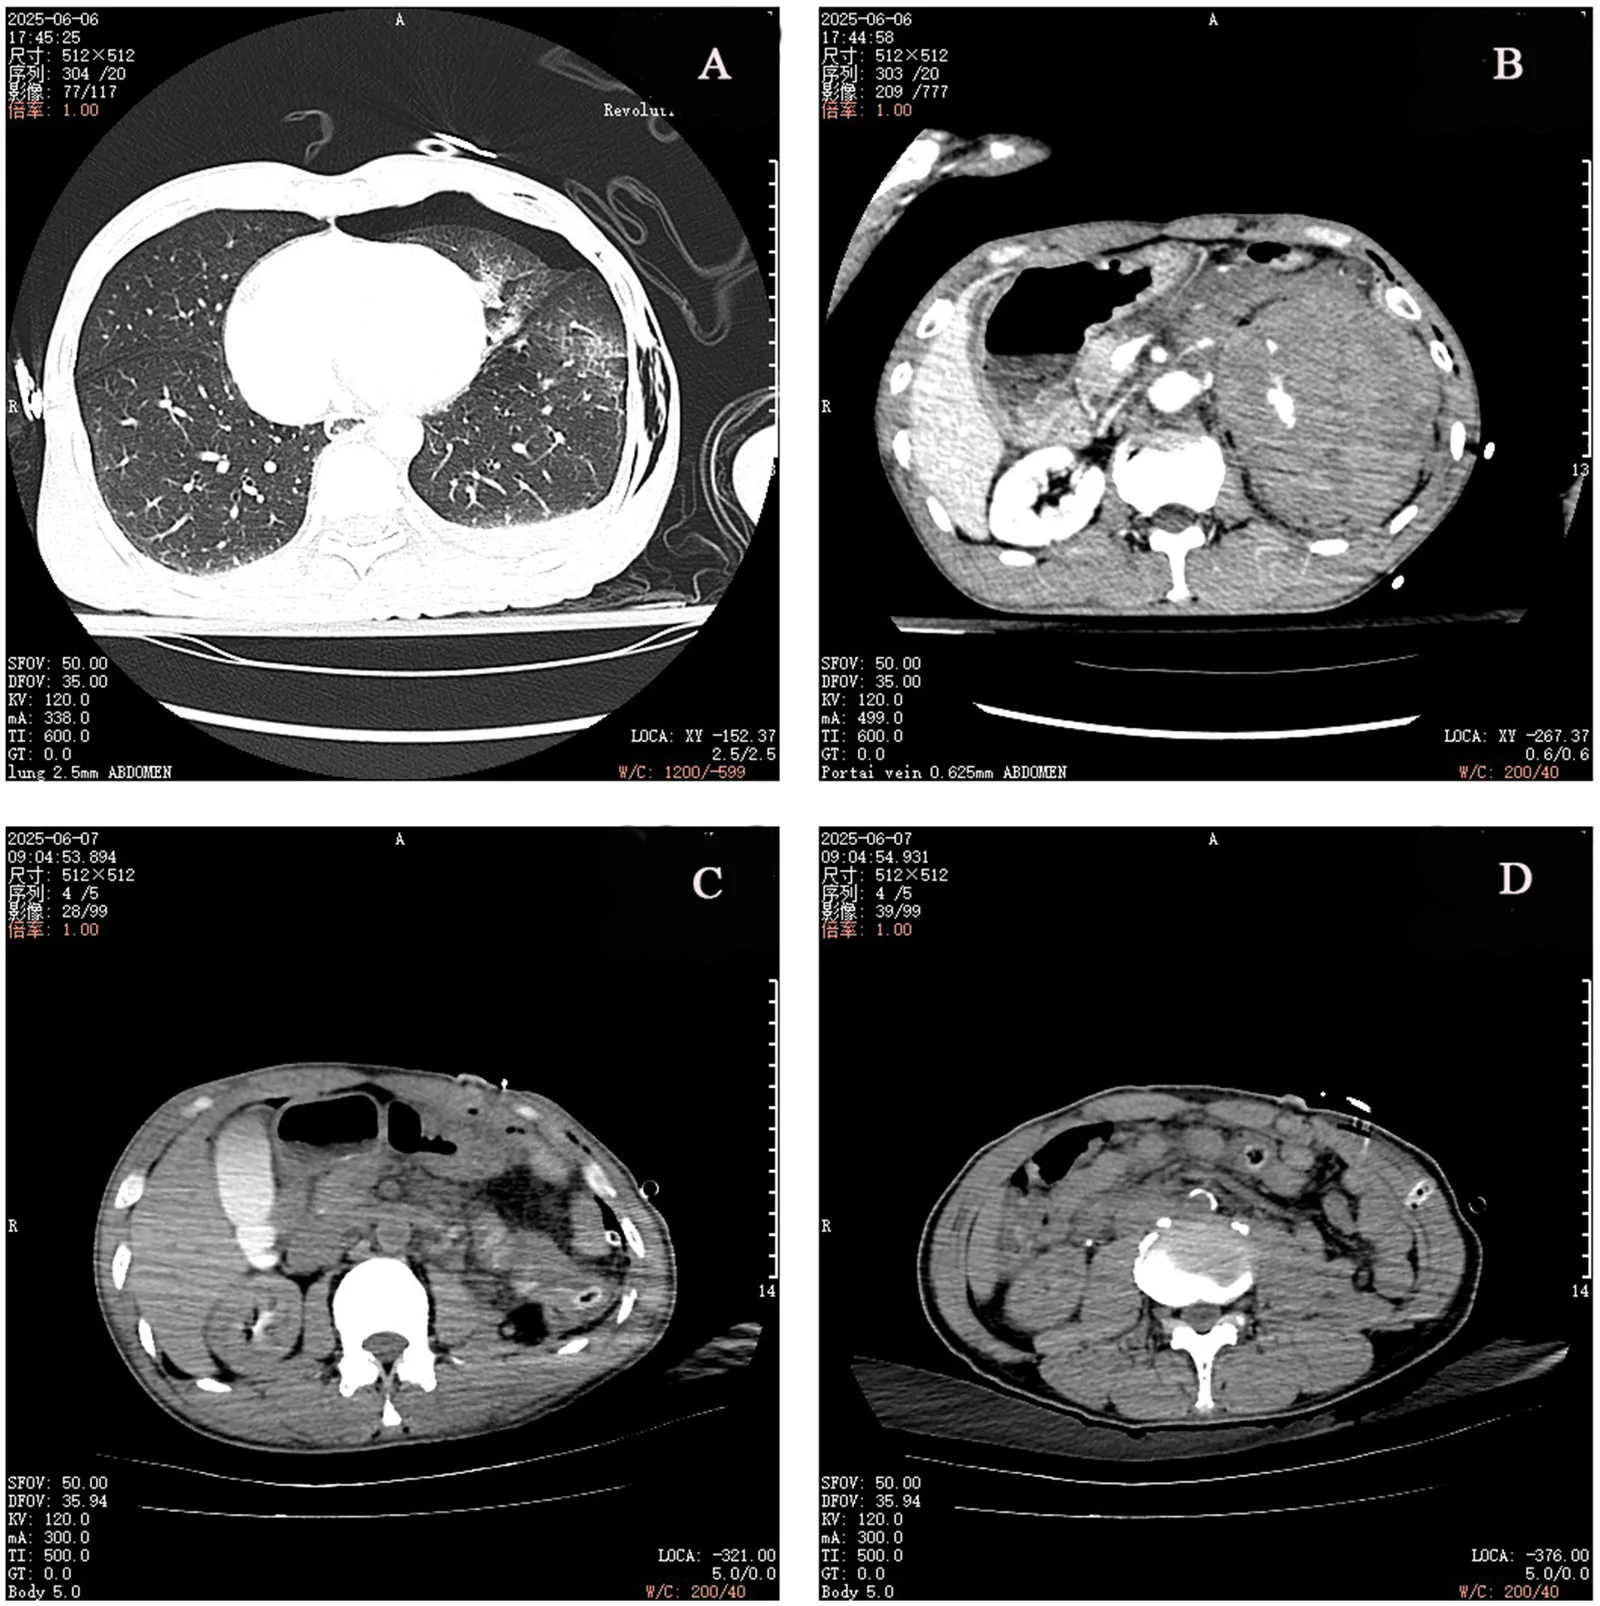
Preoperative and postoperative CT images of the chest and abdomen in Patient 2. (A) Chest CT scan reveals traumatic wet lung, hemopneumothorax, and multiple rib fractures. (B) Abdominal CT scan shows a left renal contusion with a large associated hematoma. (C, D) Follow-up abdominal CT scans after left nephrectomy demonstrate significant resolution of the hematoma compared to preoperative findings.
